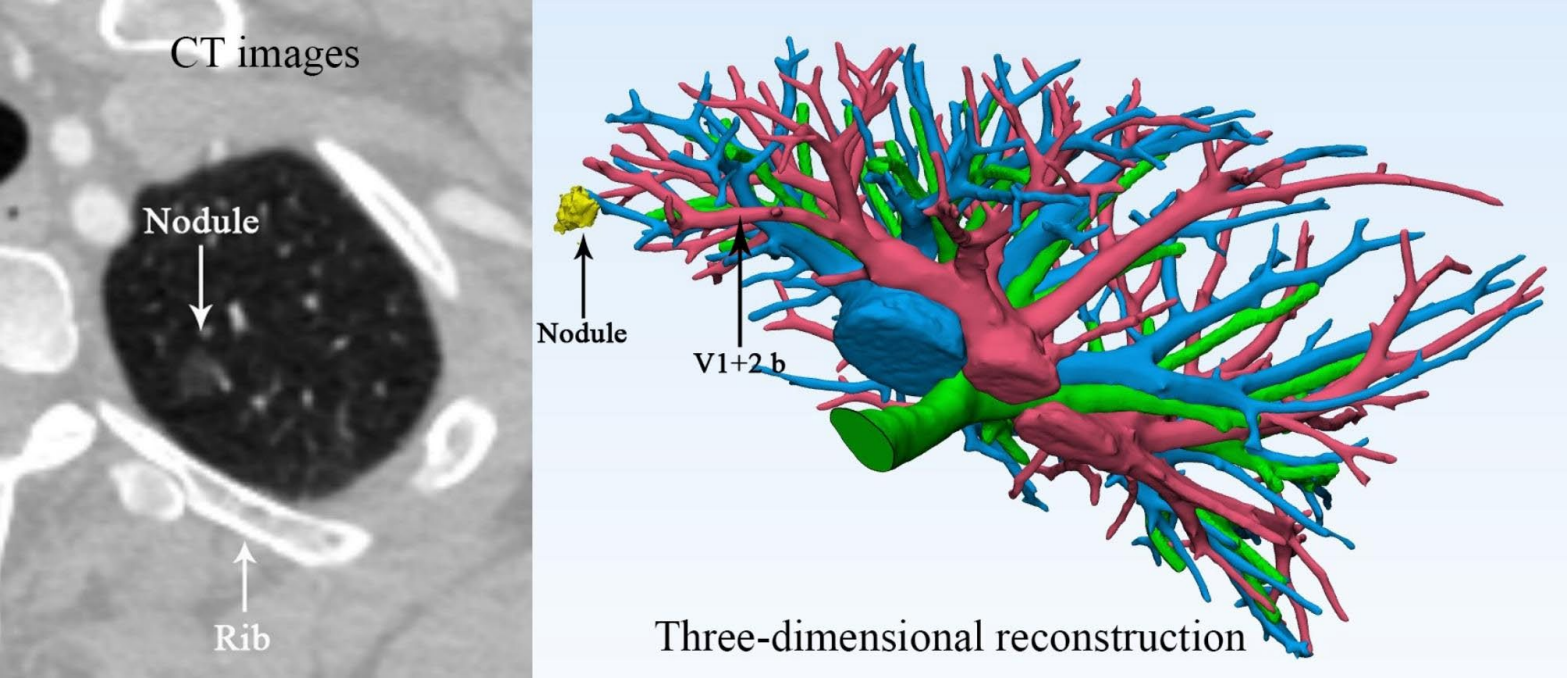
CT images of the plane with the largest diameter of the lesion and 3d reconstruction of pulmonary vessels of the patient
